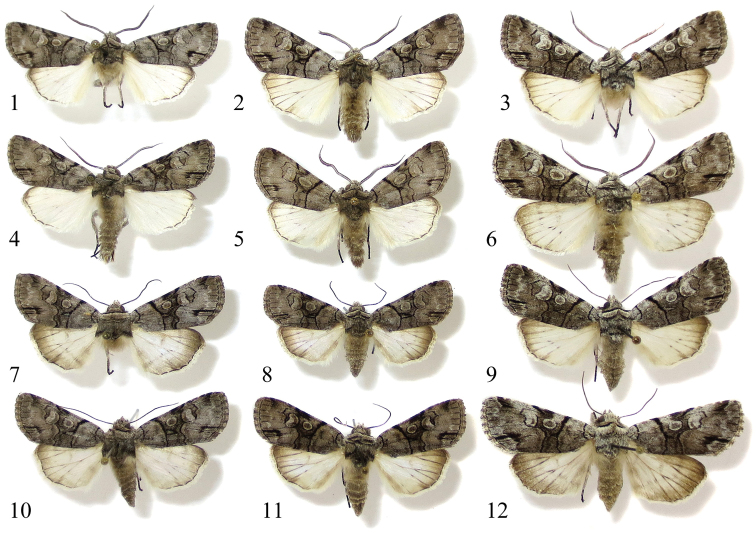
Adults of *Sympistis forbesi* and *Sympistis chionanthi*. 1 ♂ *Sympistis forbesi* HOLOTYPE, IA: Boone Co., Little Blue Stem Prairie, ex larva on *Triosteum* (UCMS) 2 ♂ *Sympistis forbesi*, IL: Champaign Co., Mahomet, ex larva on *Triosteum* (CUIC) 3 ♂ *Sympistis chionanthi*, NY: Tompkins Co., Ithaca, ex ova, reared on *Fraxinus* (CUIC) 4 ♂ *Sympistis forbesi*, IA: Boone Co., Little Blue Stem Prairie, ex larva on *Triosteum* (UCMS) 5 ♂ *Sympistis forbesi*, IL: Champaign Co., Mahomet, ex larva on *Triosteum* (CUIC) 6 ♂ *Sympistis chionanthi*, CT: Windham Co., Hampton, adult at light (UCMS) 7 ♀ *Sympistis forbesi*, IA: Boone Co., Little Blue Stem Prairie, ex larva on *Triosteum* (UCMS) 8 ♀ *Sympistis forbesi*, IL: Champaign Co., Mahomet, ex larva on *Triosteum* (CUIC) 9 ♀ *Sympistis chionanthi*, NY: Tompkins Co., Ithaca, ex ova, reared on *Fraxinus* (CUIC) 10 ♀ *Sympistis forbesi*, IA: Polk Co., ex larva on *Triosteum* (UCMS) 11 ♀ *Sympistis forbesi*, IL: Champaign Co., Mahomet, ex larva on *Triosteum* (CUIC) 12 ♀ *Sympistis chionanthi*, CT: Windham Co., Pomfret, adult at light (UCMS).
